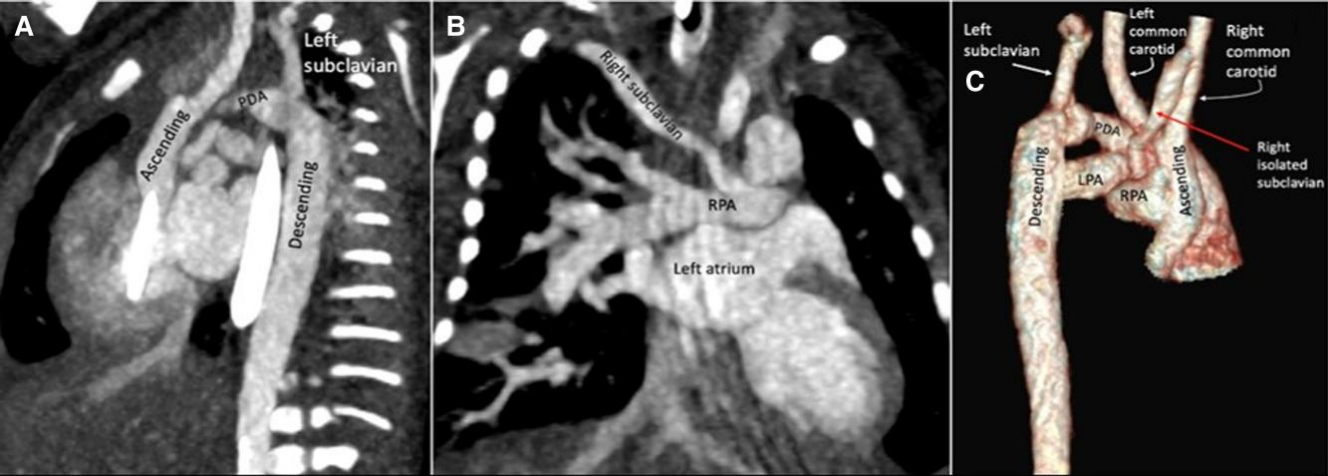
(*A* and *B*) Coronal and lateral oblique reformatted maximum intensity projection images from cardiac computed tomography angiography demonstrate an interrupted aortic arch type B and a right isolated subclavian artery. (*C*) Three-dimensional volume-rendered image in right lateral view shows the interruption occurring beyond the origin of the right and left common carotid. Left subclavian artery arises beyond the interruption. Patent ductus arteriosus continues with the descending aorta. Right isolated subclavian artery originates from the right pulmonary artery.
